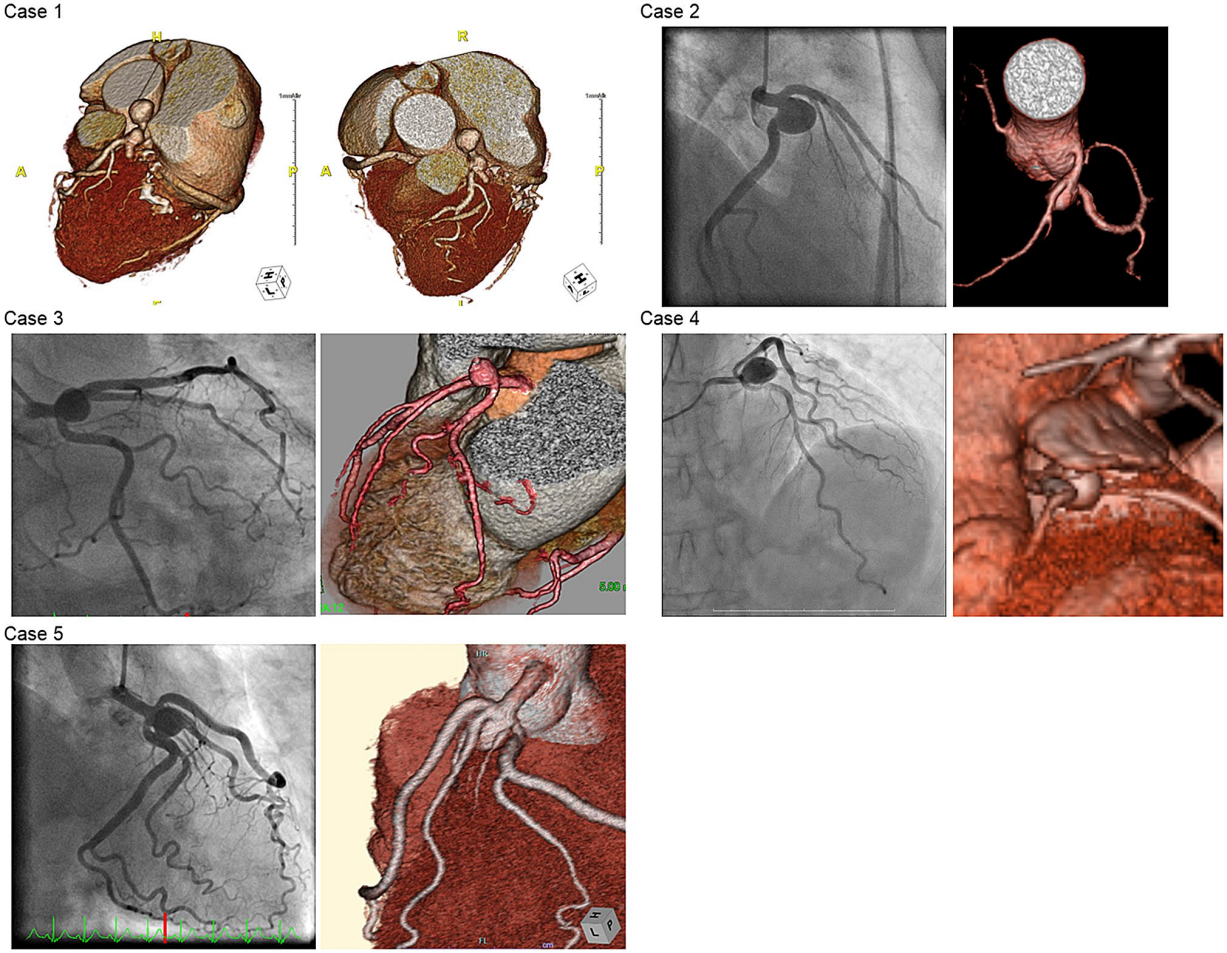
Radiological and computed tomography (CT) findings of the five patients with saccular coronary artery aneurysms
